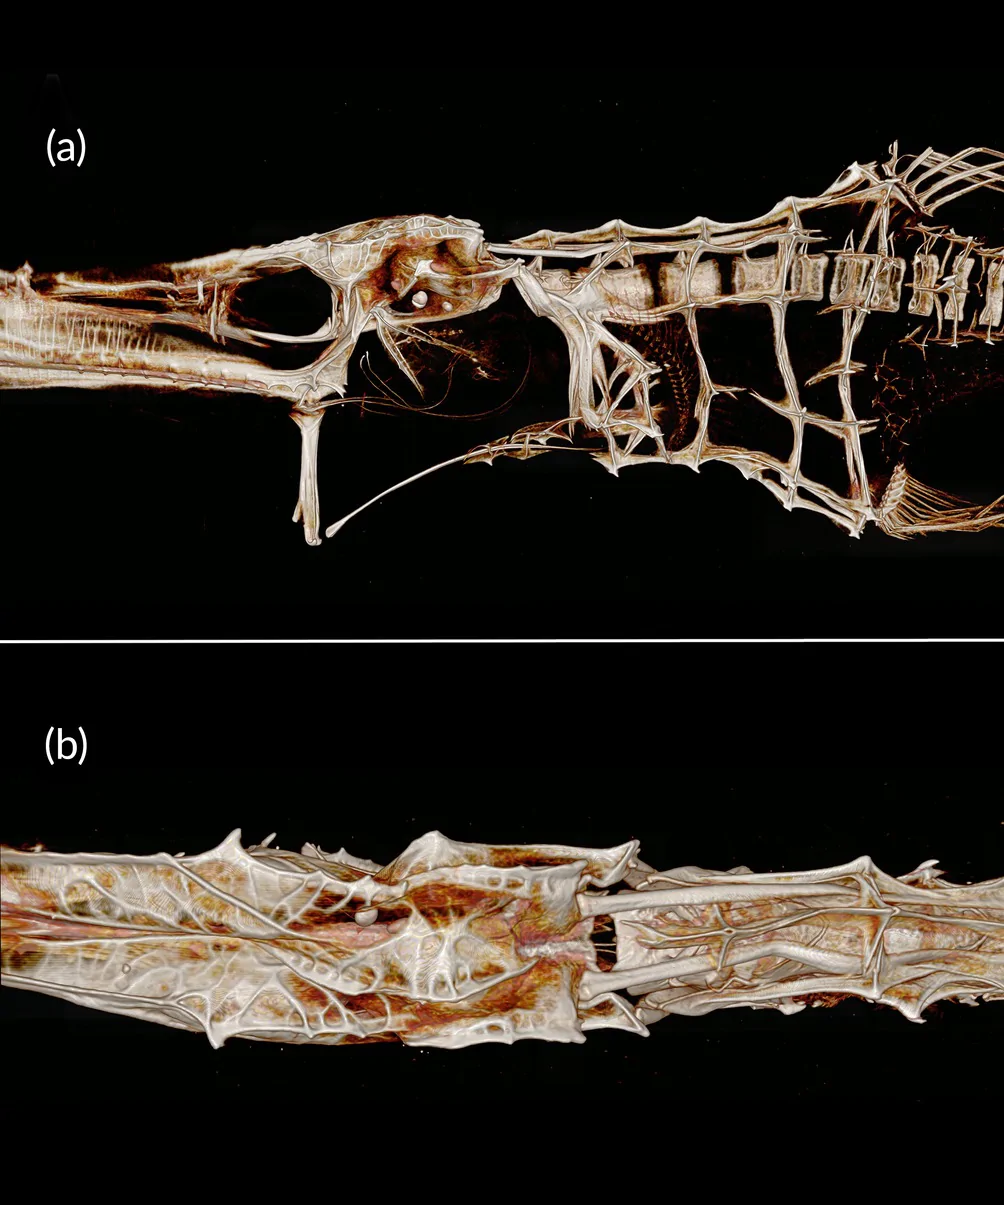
Micro‐CT reconstruction of the supraoccipital crest of CAS 240194: (a) lateral view; (b) dorsal view.
